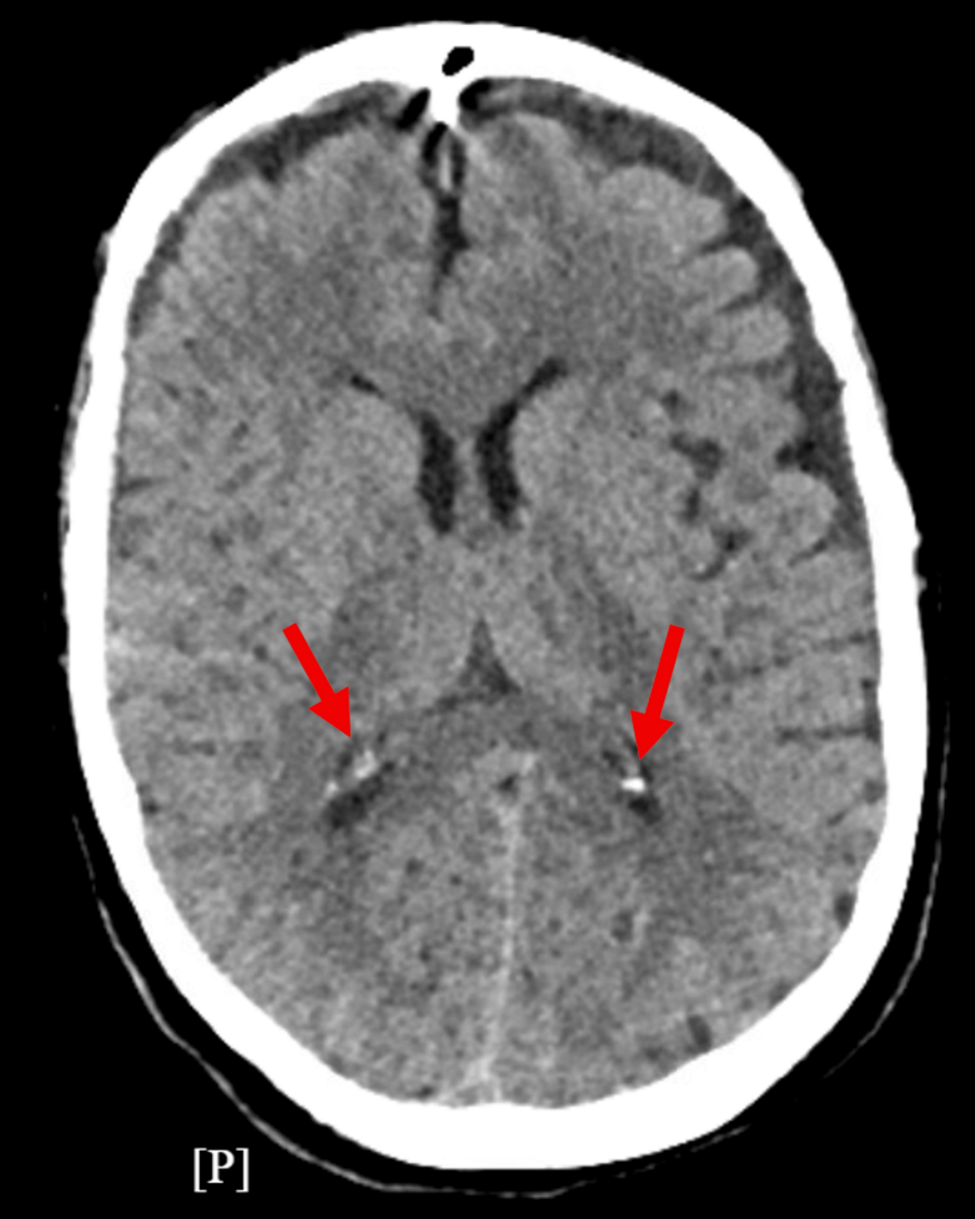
Axial non-contrast head CT demonstrates hyperdense foci concerning an acute ICH. The lobar distribution involving the cortex increases the risk of seizure activity. CT: computed tomography, ICH: intracerebral hemorrhage
